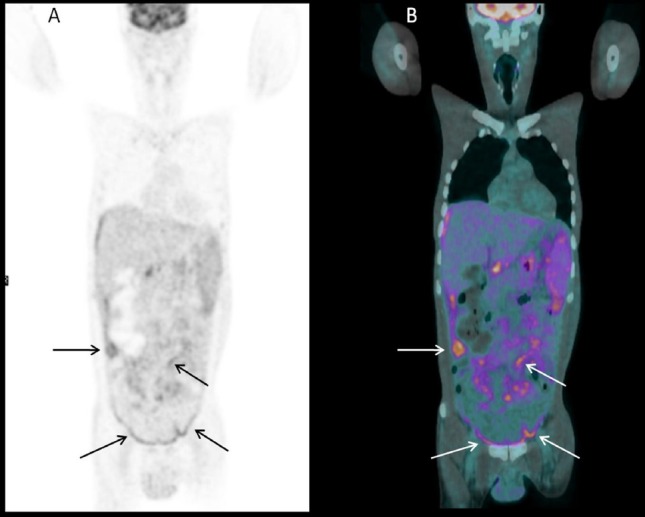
Coronal PET (A) and fused PET/CT (B) images showing high FDG uptake at the abdominal and pelvic peritoneal cavity. Black and white arrows indicate the most intense sites of tracer uptake.
